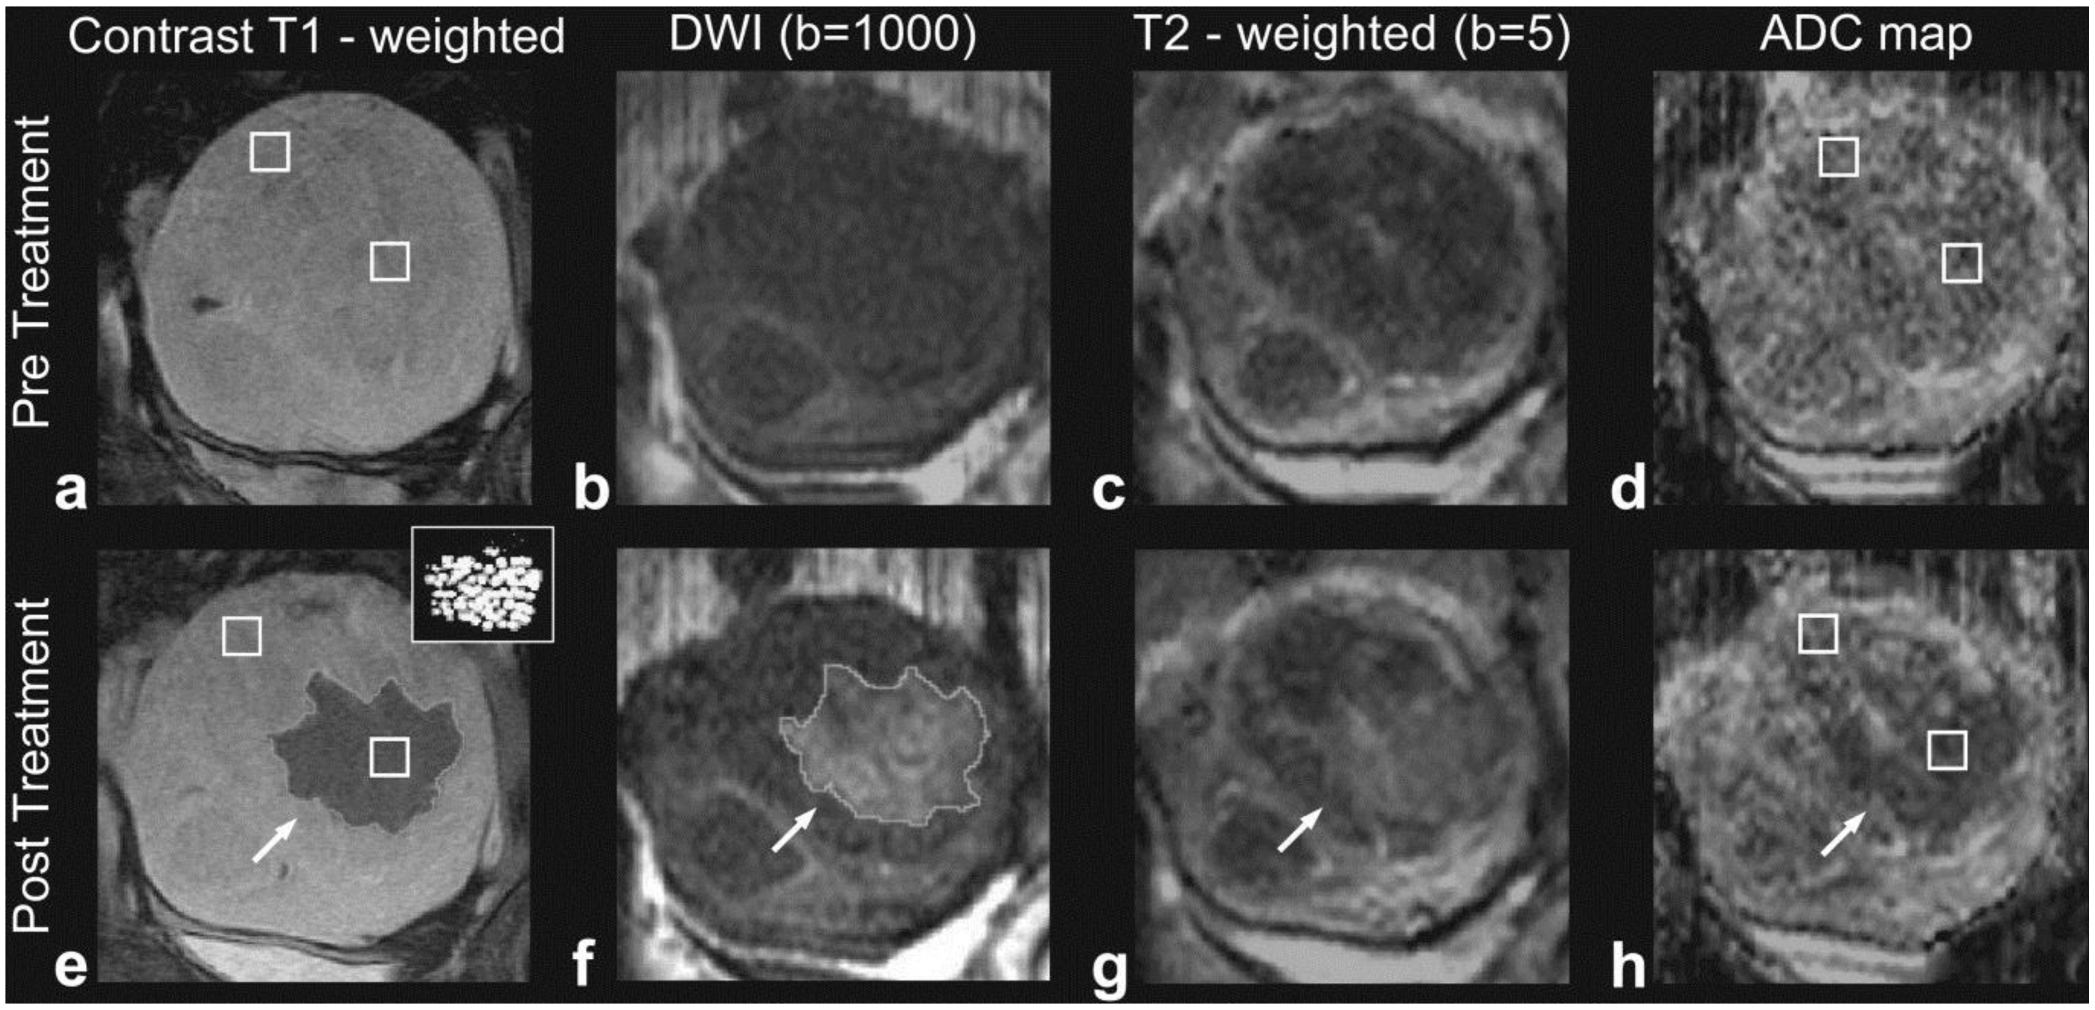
Multiple parameter MRI assessment of thermal HIFU ablation of uterine fibroids. Patient 31 (52 years old, intramural leiomyoma). Coronal images at the treatment plane pre- and post-HIFU ablation. (a) Contrast-enhanced T1-weighted image acquired pretreatment. (b) DW image acquired pretreatment. (c) T2-weighted image acquired pretreatment. (d) ADC map pretreatment (ADC value: 1287 ± 258 × 10^−6^ mm^2^/s). (e) Contrast-enhanced T1-weighted image acquired posttreatment. The area of the resulting nonperfused region (demarcated with a light grey line in pane e) was 20.8 cm^2^. The thermal dose estimate calculated from the temperature maps is shown in the inset figure, at the upper right corner. Areas that reached a thermal dose of 240 min are grey, and areas that reached a thermal dose of 18 min are white. The area of the region with a thermal dose exceeding 18 min at 43°C was 14.7 cm^2^. (f) DW image acquired posttreatment. The area of the resulting hyperintense region (outlined with a light grey line in pane f) was 22.8 cm^2^. (g) T2-weighted image acquired posttreatment, the affected area appears hyperintense (h) ADC map posttreatment, the affected area appears hypointense (ADC value: 923 ± 175 × 10^−6^ mm^2^/s). Figure adapted from [8].
